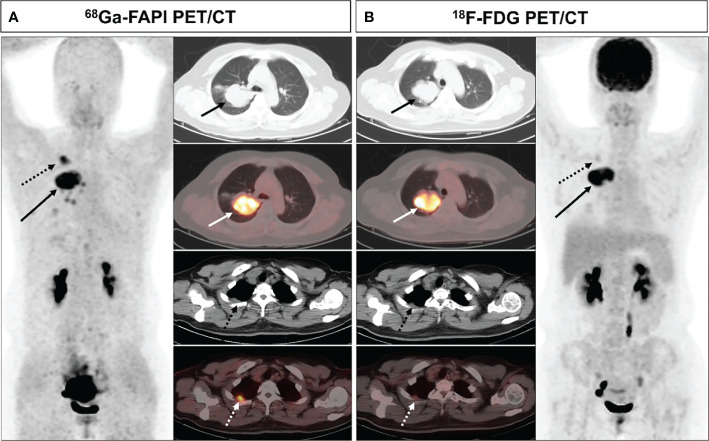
A 44-year-old female (patient 1) diagnosed with adenocarcinoma. ^68^Ga-FAPI PET/CT (A) revealed increased FAPI uptake in the primary lesion (solid arrows, SUVmax = 11.7) and pleural lesion (dashed arrows, SUVmax = 7.0). ^18^F-FDG PET/CT (B) also showed high FDG uptake in the primary lesion (solid arrows, SUVmax = 12.4), while the pleural lesion with only mild FDG uptake (dashed arrow, SUVmax = 2.2). The pleural lesion was deemed likely to be metastatic, as confirmed upon subsequent follow-up.
